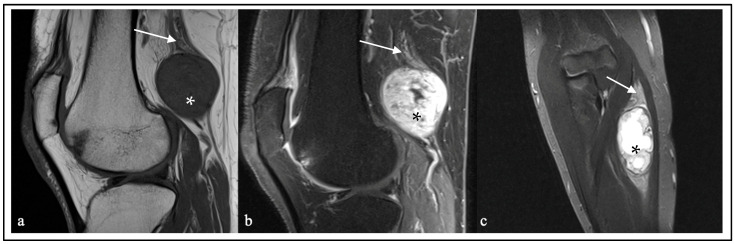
MRI features of hybrid nerve sheath tumors. (a) T1 MRI shows a homogeneous hypointense (*) mass associated with the sciatic nerve (arrow). There is no evidence of infiltration into adjacent tissues or a perilesional edema. (b) A heterogeneous contrast enhancement of the mass (*). (c) T2 STIR sequence shows an inhomogeneous, partially cystic mass (*) originating from the cutaneous antebrachial medial nerve (arrow).
